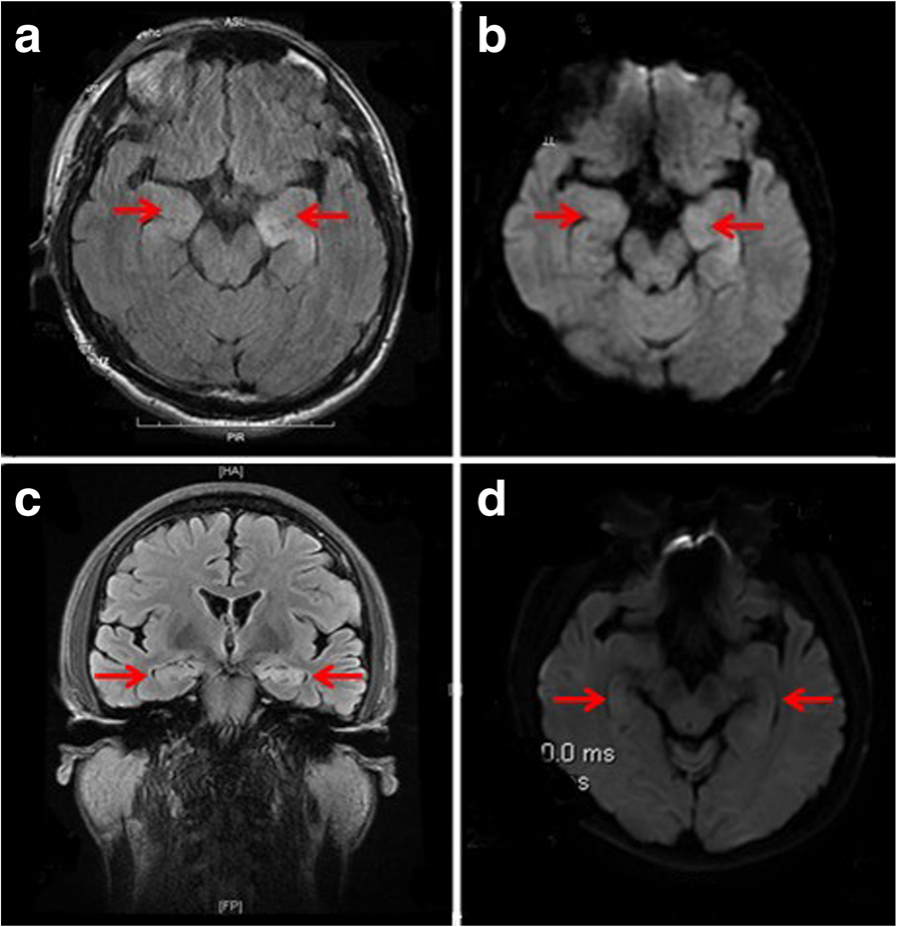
Cranial magnetic resonance images (MRI) of this patient. T2-weighted fluid-attenuated inversion recovery (a) and the corresponding plane in diffusion weighted imaging (b) sequences showed hyperintensities of bilateral hippocampus. Repeated MRI showed some abnormal hyperintensities particularly in the left hippocampus 12 days after the initial MRI scan (c, d)
